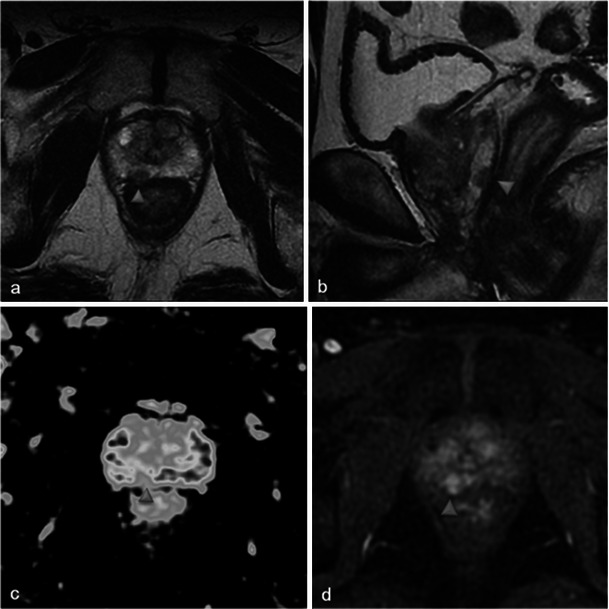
Pre-treatment MRI of a 65-year-old patient with a PSA level of 9.7 ng/ml and a positive biopsy in the right prostate apex (adenocarcinoma Gleason 6). Axial and sagittal T2-weighted images (a, b) show a hypointense nodule in the right peripheral prostate with restriction on the apparent diffusion coefficient map of the diffusion-weighted image (c, low signal intensity) and early enhancement on the axial dynamic contrast-enhanced MR image (d, *arrowhead*)
